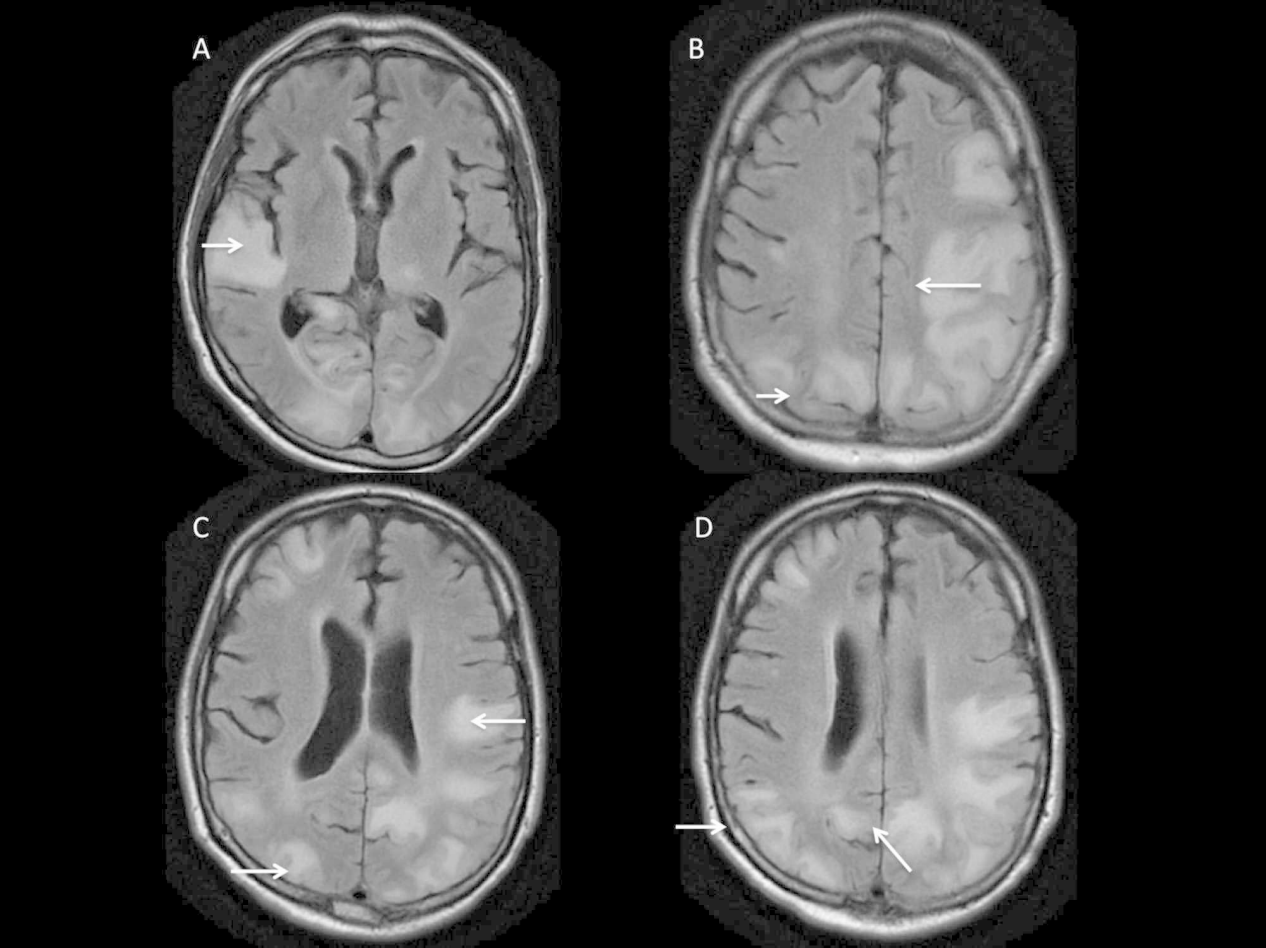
T2 - FLAIR MRI of brain. MRI of brain (A-D) demonstrates the development of new multifocal areas of enhancement (arrows) suggestive of new ischemic lesions in multiple vascular territories.
